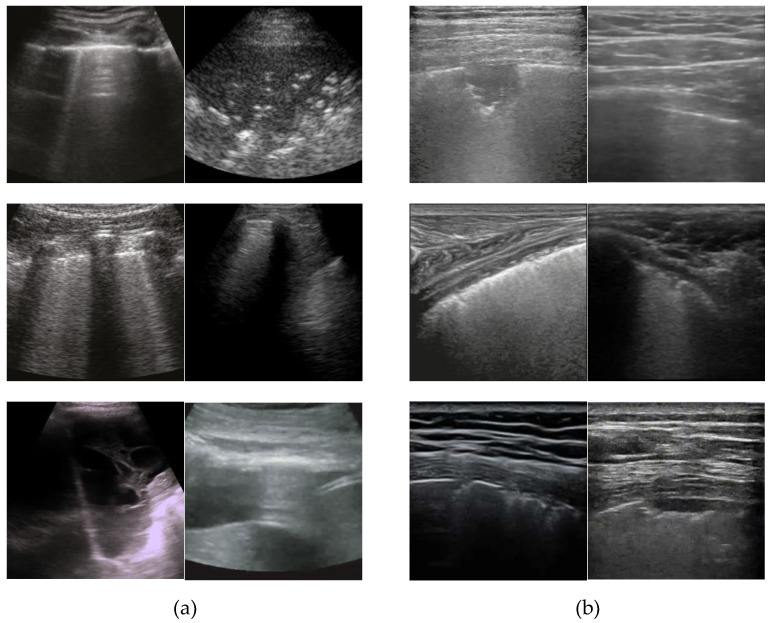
Examples of ultrasound images obtained from different probes and records using (a) a convex sensor and (b) a linear sensor.
